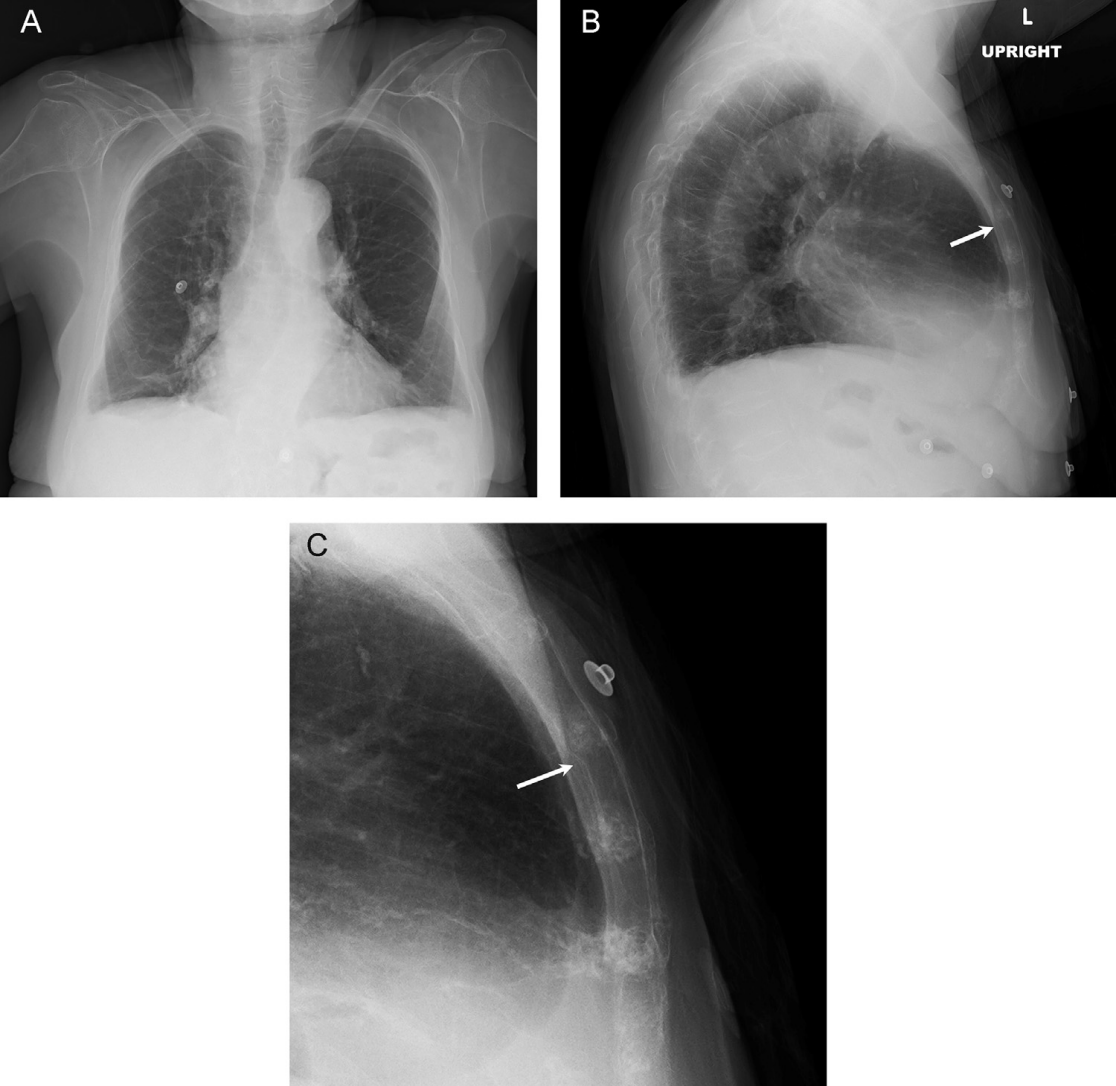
(A) PA radiograph of the chest shows no acute pulmonary abnormality. (B) Lateral radiograph of the chest also shows no acute pulmonary abnormality. Exaggerated thoracic kyphosis and multiple thoracic compression fractures are seen. There is subtle cortical offset at the posterior margin of the sternum (arrow). (C) Magnified view of lateral radiograph of the chest confirms cortical step-off at the posterior margin of the sternum (arrow). This is concerning for a nondisplaced sternal fracture.
